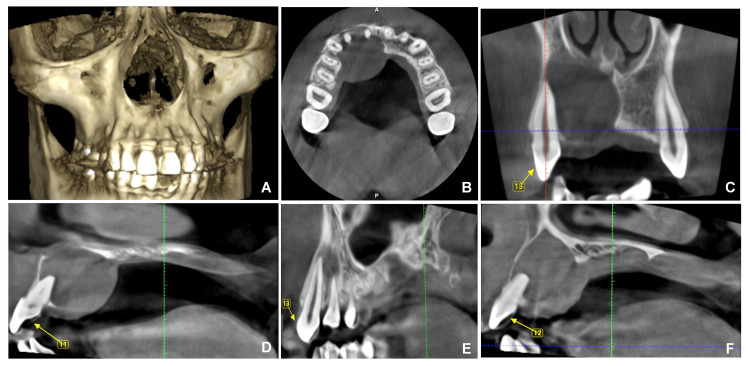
CBCT of the patient. A: Three-dimensional reconstructed image of CBCT; B: axial section; C: coronal section with 13; D: sagittal section with 11; E: sagittal section with 13; and F: sagittal section with 12. CBCT: cone-beam computed tomography. Image Credit: Prasanna Sonar.
